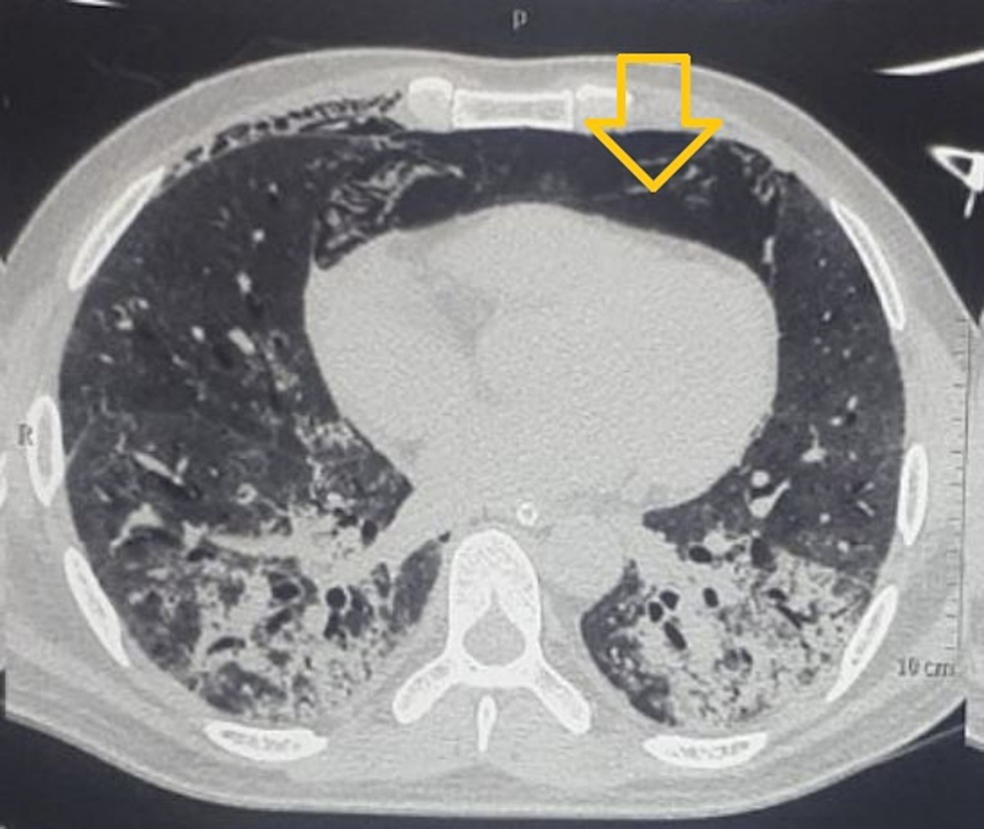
Transverse slice of CT image from mid-thoracic level showing pneumomediastinum (shown by orange arrow)
